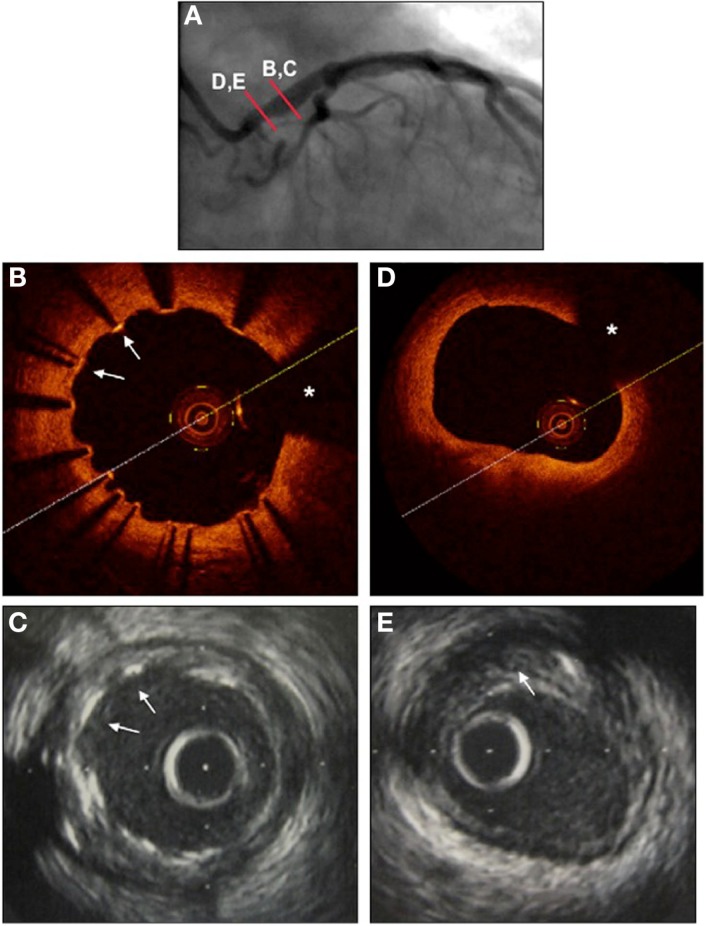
Left main coronary artery imaged with angiography (A), optical coherence tomography (B,D), and intravascular ultrasound (C,E), 1 year following stenting using a drug-eluting stent. (A) Coronary angiography RAO view. Proximal mild left main lesion and dilated stent in mid and distal portion of left main coronary artery. (B) OCT to mid left main. Stent struts are seen well-apposed to the vessel wall, with precise detail (arrows). Wire artifact shadow marked as asterisk. (C). IVUS to mid left main at same site. Stent struts are seen, and appear well-apposed, but without the precision of the OCT images (arrows). (D) OCT to ostium of left main. The lumen is very clearly seen (area calculated at 7.1 mm^2^), but no detail is provided as to the nature or extent of underlying atherosclerotic plaque. Wire artifact shadow marked as asterisk. (E) IVUS to ostium of left main at same site. The lumen is well visualized (lumenal area calculated at 6 mm^2^). The extent of underlying atherosclerotic plaque is evident, marked with arrow (calculated at 40% area stenosis).
